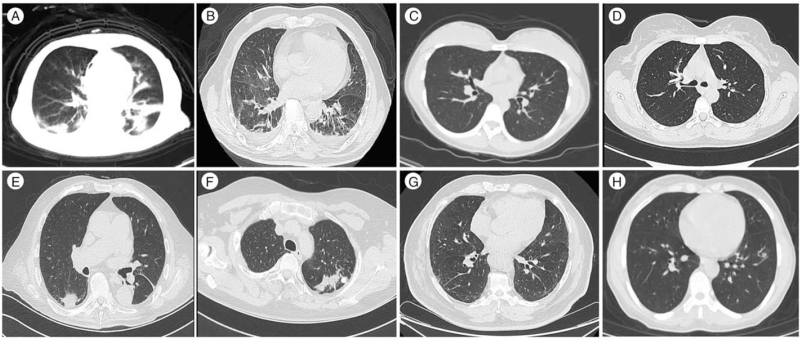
Chest CT scans for all 8 patients. (A and B) Case 1 and 2 had bilateral patchy consolidation and multiple ground-glass opacities. (C) Case 3, (D) Case 4, (E) Case 5, (F) Case 6, (G) Case 7, (H) Case 8.
